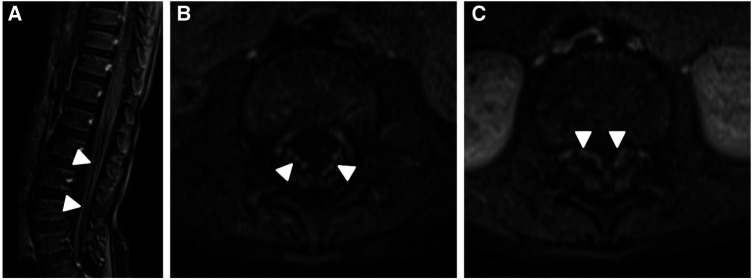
Contrast enhanced MRI of the lumbar spine obtained at the same time of the brain MRI shows smooth enhancement of the cauda equina nerve roots, particularly the anterior nerve fibres (white arrowheads in A–C).
